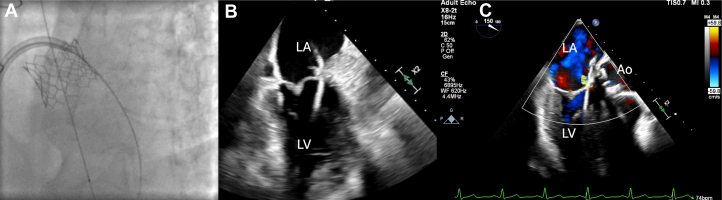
Crossing the Fistula (A) Angled glide wire advanced through the fistula via transseptal puncture and LA on fluoroscopy. (B and C) TEE view of angled glide wire through the fistula into the LV. TEE = transesophageal echocardiographic; other abbreviations as in Figure 2.
